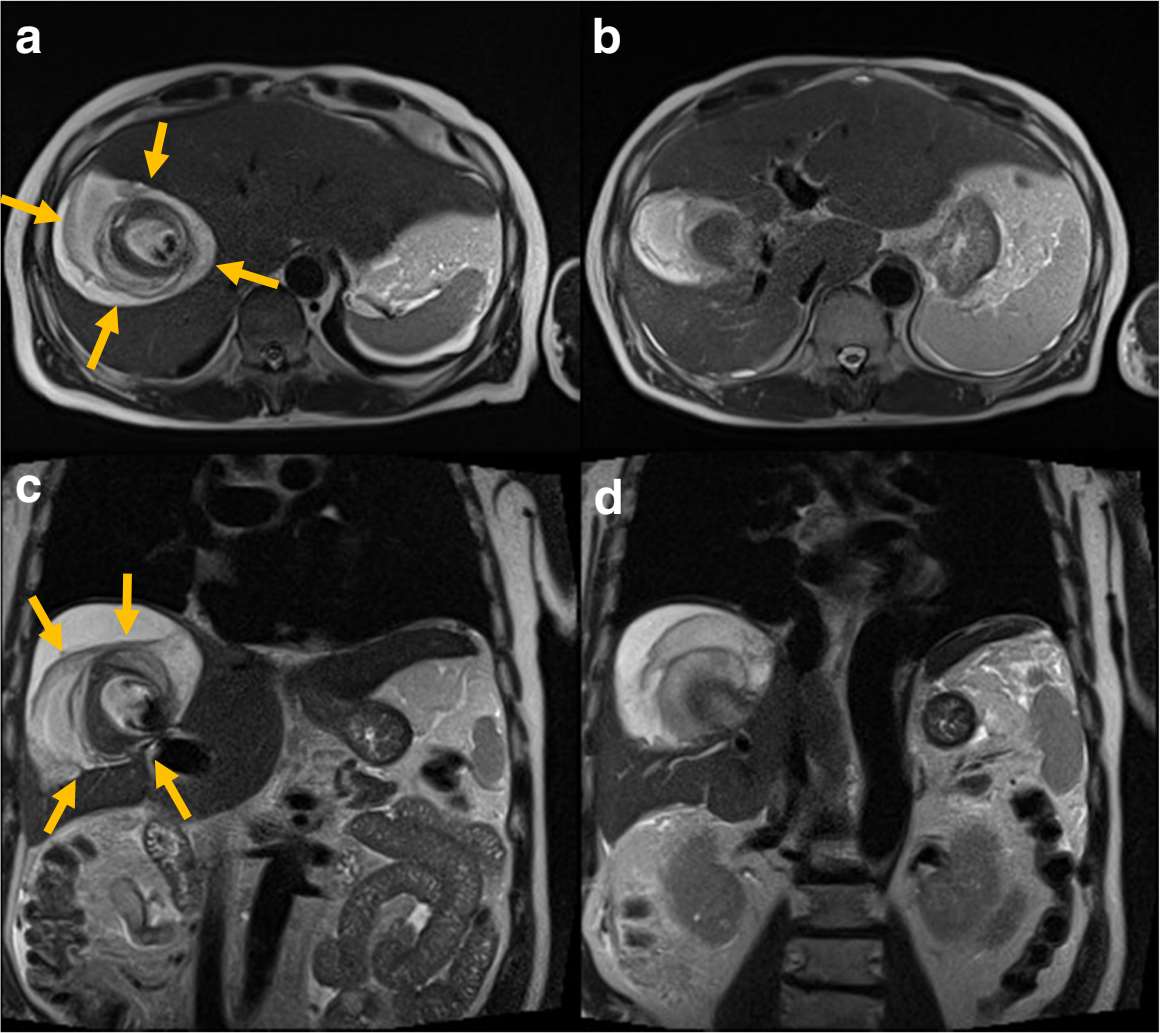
Dynamic MRI findings 3 months after surgery. a, b Axial images of dynamic MRI. c, d Coronal images of dynamic MRI. An arterioportal fistula and portal vein aneurysm were incidentally detected. Layers of old and subacute hematomas were clearly observed, and these layers surrounded the aneurysm (arrows). We suspected a pseudoaneurysm based on these MRI findings
